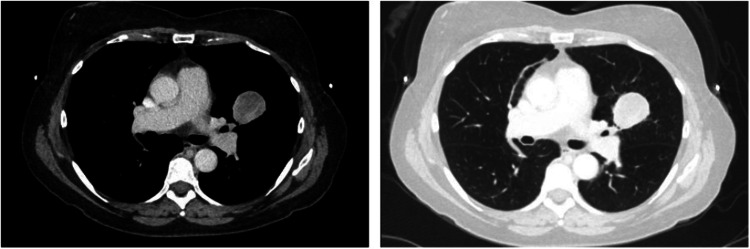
Chest CT scan: a new mass in the left upper lobe that measured 3.2 cm × 3.8 cm
